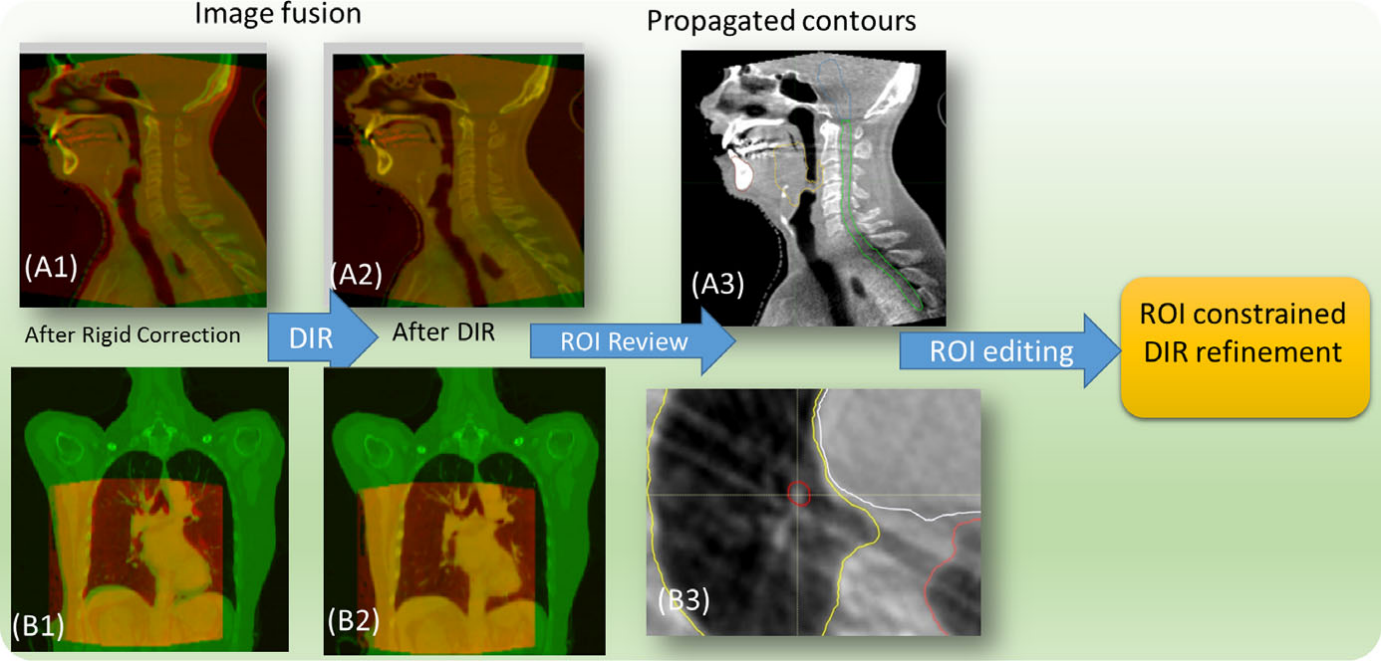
Qualitative inspection of CBCT–CT deformable registration by fusion images and propagated contours: CT in green and CBCT in red, A1–A3 head and neck patient, B1–B3 phase‐matching lung CBCT.
